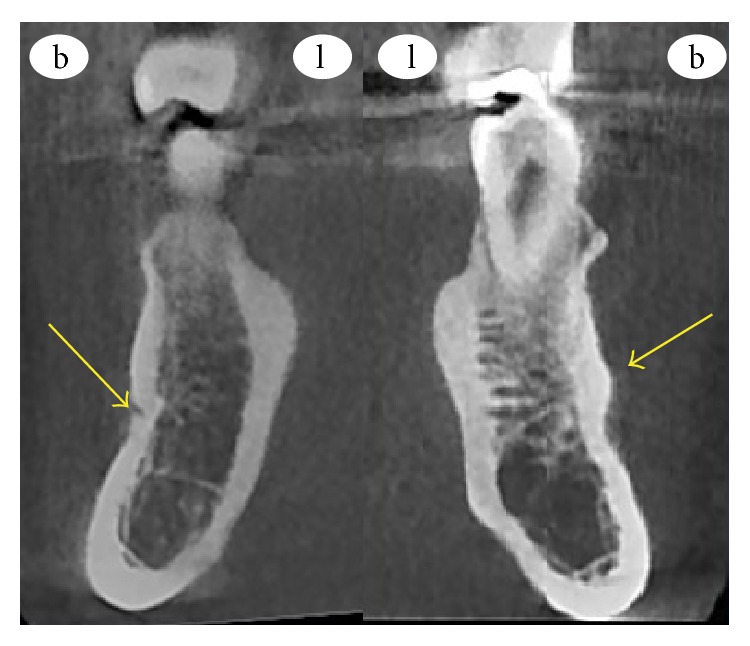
Coronal CBCT slice in which the openings of the right MF and the left protuberance at the MF area are pointed by the arrows.
